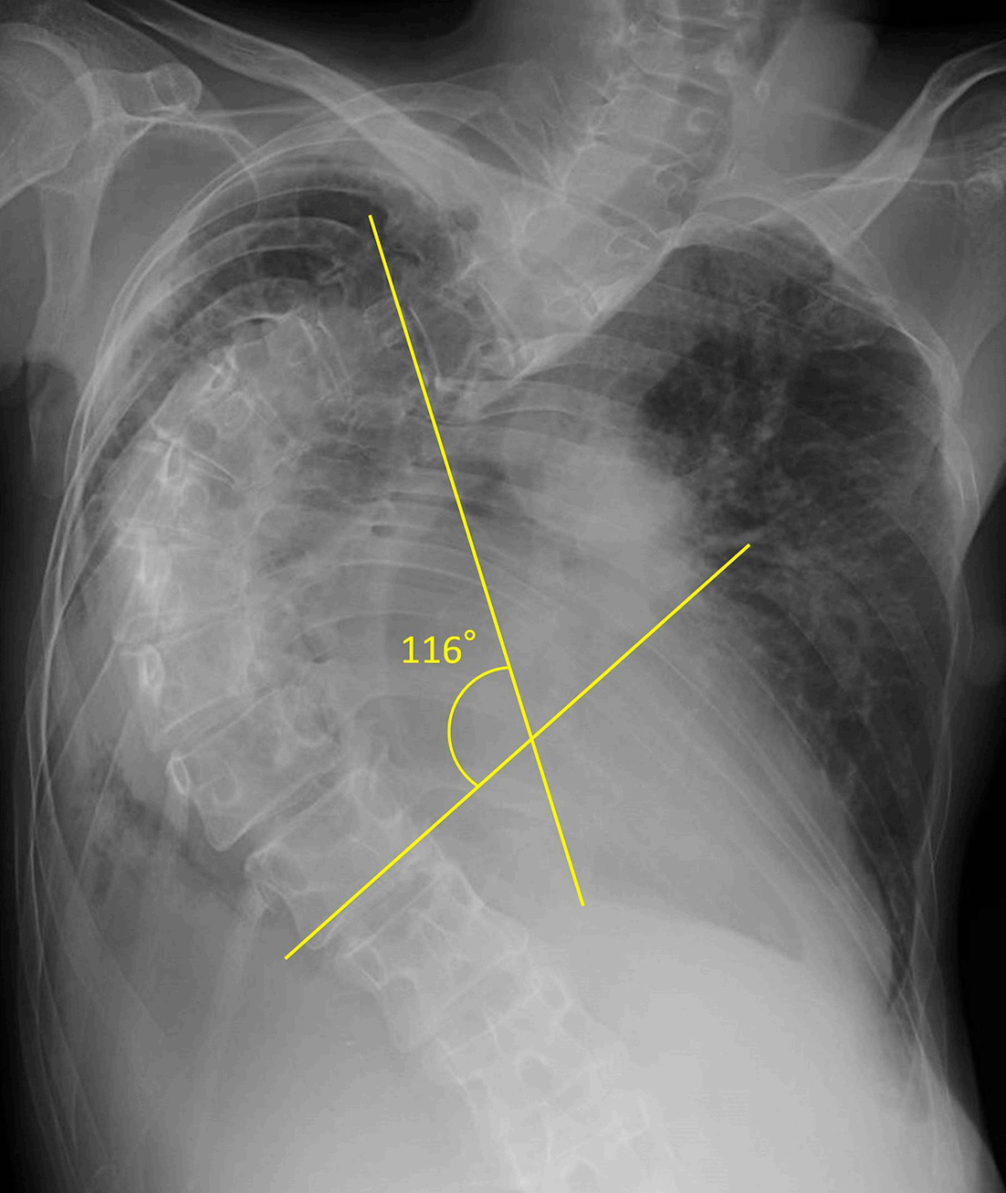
Chest radiography depicting severe scoliosis (Cobb angle, 116°).
